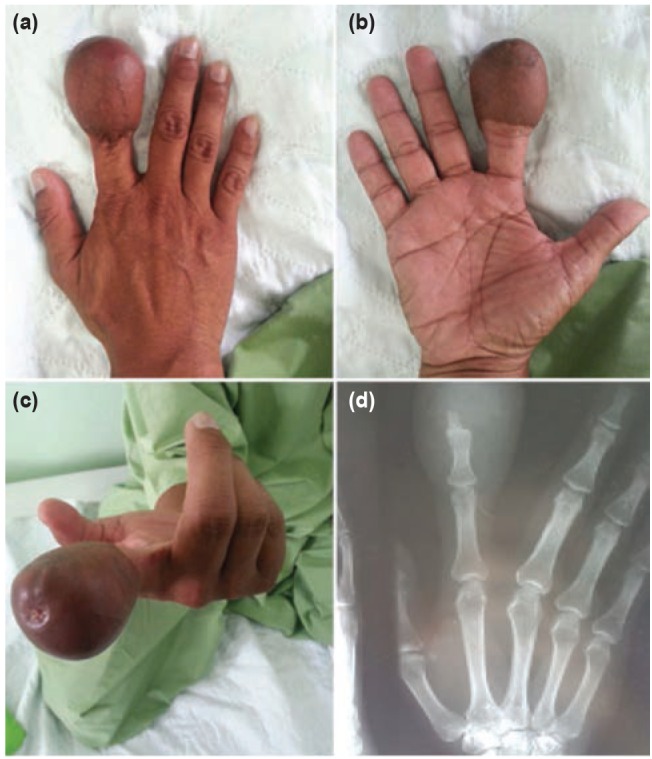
Preoperative images of (a) Dorsal, (b) Volar, (c) Fingertip and (d) Radiograph of the index finger with bulky soft tissue from previous skin flap.
